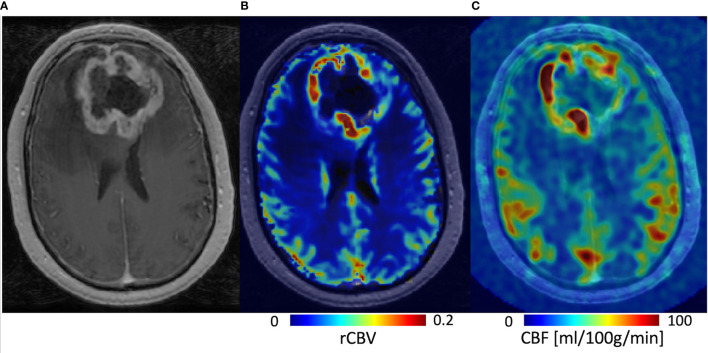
Three-months post-radiotherapy follow-up in a 58-year-old patient diagnosed with a glioblastoma, IDH-wildtype, studied on a 3T GE MRI system. (A) The post-GBCA *T*_1_-weighted series shows thick rim enhancement, which could theoretically also be pseudoprogression after recent completion of standard-of-care radiotherapy and concomittant temozolomide. (B) The DSC rCBV map, acquired with 2D EPI (TE/TR = 45/1500 ms), indicates general hyperperfusion of the enhancing outer tumor rim, which makes early true progression more likely and is thus decisive for further therapy planning. (C) The ASL CBF map, which was acquired with 3D pCASL using white paper settings, shows hyperperfusion concordant with the DSC rCBV map but without the need of gadolinium administration.
